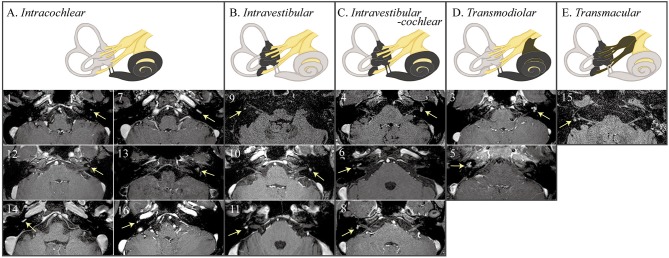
MRIs of the patients with intralabyrinthine schwannoma (ILS) according to Kennedy classification. 3D contrast-enhanced T1 or fluid attenuated inversion recovery (FLAIR) images show nodular enhancements within the labyrinth (arrows).
